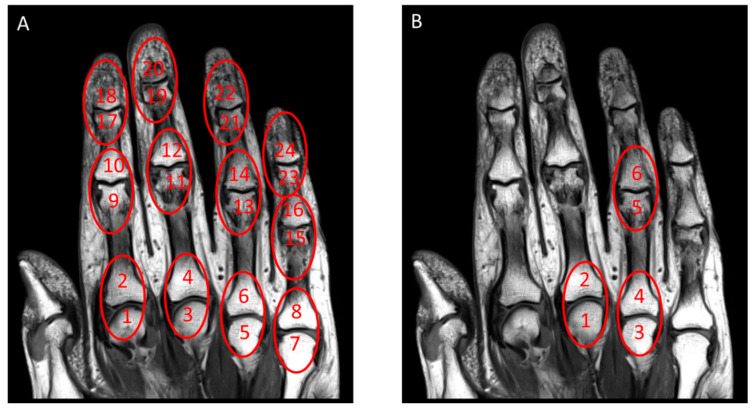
Joint regions and sub-regions in Psoriatic Arthritis Magnetic Resonance Imaging Score (PsAMRIS) versus simplified (s) PsAMRIS. Coronal T1-weighted MR image of a representative patient suffering from psoriatic arthritis (PsA). (A) In the full PsAMRIS, inflammatory and destructive changes associated with PsA are assessed in distinct regions and subregions of the hand. Circles indicate scored joints (i.e., regions), while numbers indicate joint sites (i.e., subregions). (B) Simplified (s) PsAMRIS. In A, 24 joint sites and/or 12 joints were evaluated, while in B, this number is reduced to 3 joints and/or 6 joint sites that were determined to be most responsive to clinical change.
